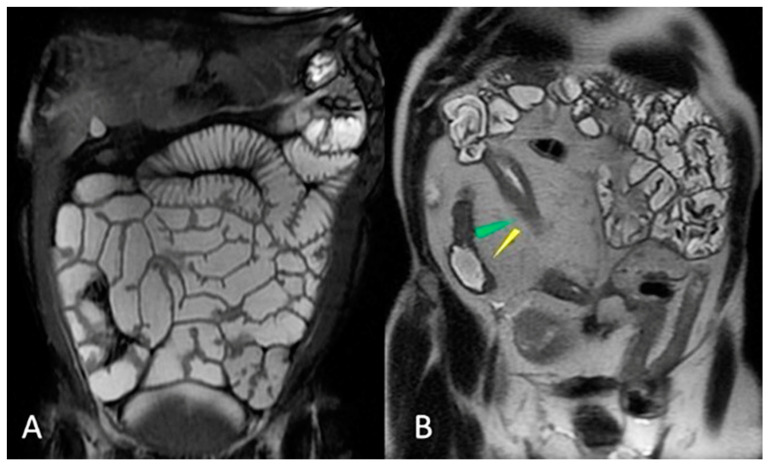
Coronal (A) FIESTA sequences do not show fibrofatty proliferation of normal mesenteric fat with kissing bowel loops. Coronal T2-weighted images (B) show fibrofatty proliferation (creeping fat), i.e., hypertrophy of the mesenteric fat, which separates bowel loops as a sign of mesenteric inflammation in Crohn’s disease. Affected bowel loops are separated by focal/regionally increased fat (fibrofatty proliferation or creeping fat): Opposed green and yellow arrowheads in (B).
